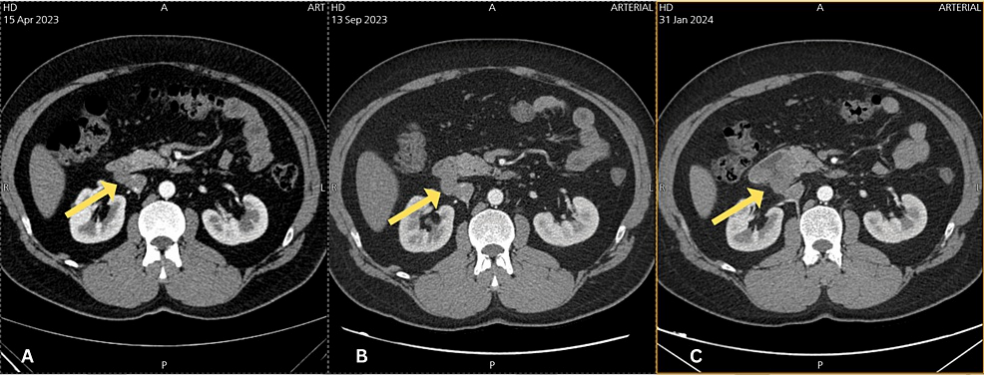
Coronal view of multiple CT scans with IV contrast showing the progressively enlarging mass (yellow arrows) (a) In April 2023, a single enlarged paracaval lymph node was seen and measured about 12 mm in maximum short axis. (b) In September 2023, the lymph node had showed an 18% increase in its size and measured about 2×1.6 cm. (c) In January 2024, a newly appearing soft tissue like lesion involved the lumen of the second part of the duodenum and it was inseparable from the paracaval lymph node.
